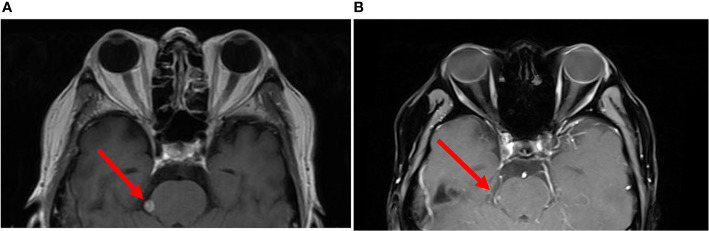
Brain imaging of the patient before and after CART treatment. (A) Brain MRI immediately before CART infusion. (B) The same region on day 30 after CART infusion. Red arrows indicate the location of one of the intracranial abnormalities. The patient was discharged on day 24, and panel (B) was done using a different MRI instrument in a local hospital in the patient’s hometown.
